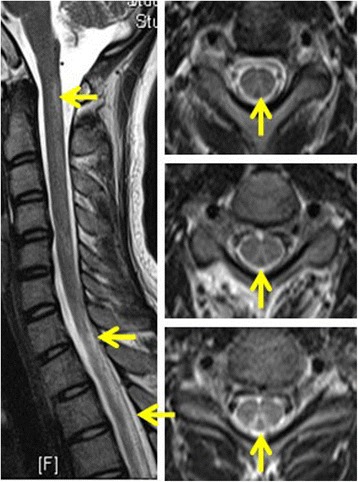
The MRI of spinal cord disclosed abnormal hyperintensities within the dorsal cervical spinal cord. On axial series, V-shaped T2 hyperintensities were again noted within the dorsal cervical spinal cord
